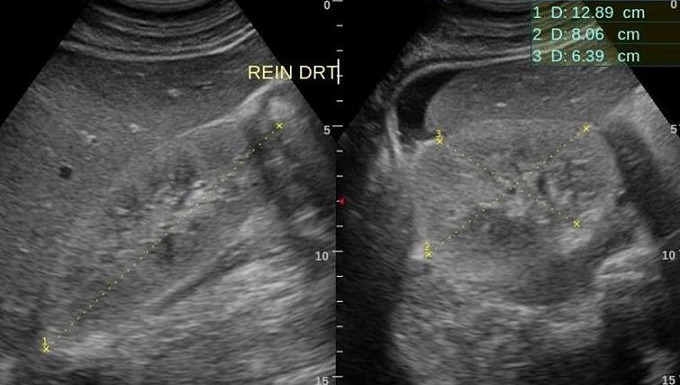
Ultrasound of the right kidney showing characteristic lesions of HIV-related nephropathy (HIVAN): increased size, maintained cortico-medullary differentiation and marked echogenicity
